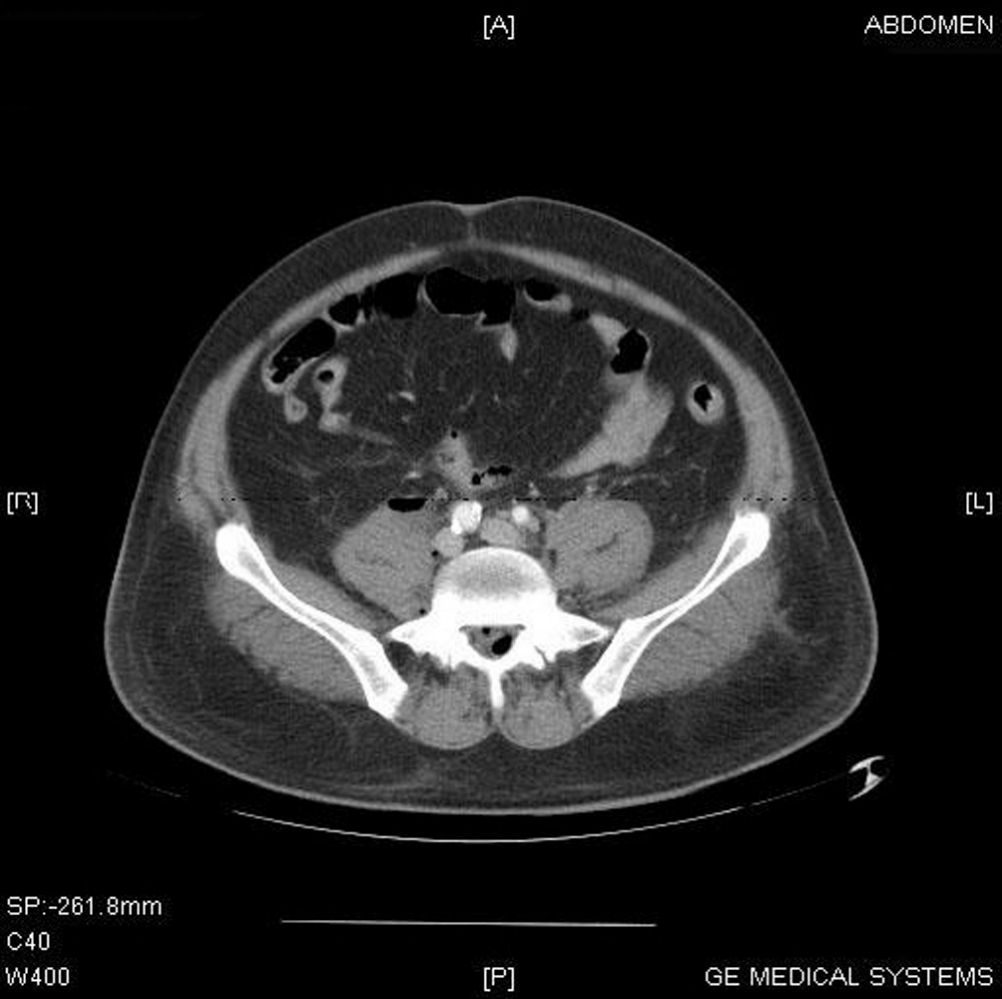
CT of the abdomen demonstrating extensive air and fluid involving the fascias along the psoas muscles, and air along the epidural space of the spinal canal in the lumbar region.
